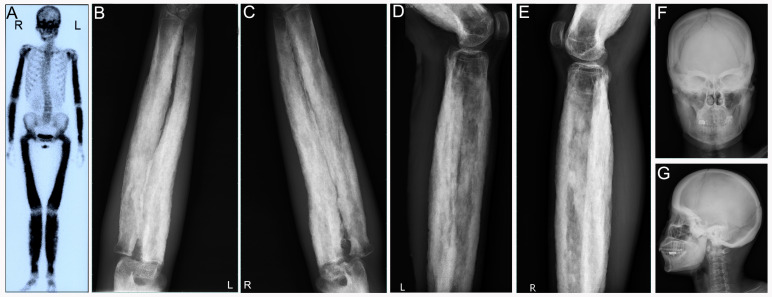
Patient 4: (A) ${}^{99m}$Tc-MDP bone scan demonstrated symmetric uptake of radiotracer mainly in the skull, upper and lower extremities. Radiographs of both upper limbs (B,C) and lower limbs (D,E) revealed thickened cortices and narrowed medullary space. (F,G) X-ray films of anteroposterior position and lateral position showed the skull of the patient was thickened as well.
